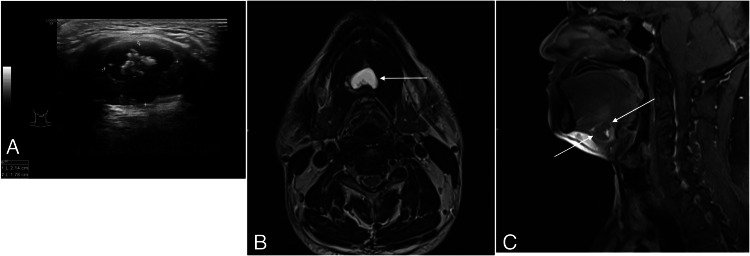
(A) US transverse image through the right mid thyrohyoid region shows a well-defined complex cystic lesion with a thick irregular wall and internal bright echogenic content which shows posterior shadowing. (B) Axial T2-weighted MRI of the neck shows a well-defined complex cystic lesion in the mid upper neck anterior to the thyrohyoid region with involvement of floor of mouth. (C) Sagittal T1 fat saturated post contrast (gadolinium) MRI of the neck shows a complex cystic lesion in the upper neck anterior to the thyrohyoid region with involvement of the floor of the mouth. It shows an enhancing thick irregular wall and a posteriorly situated enhancing nodule. US: Ultrasound, MRI: Magnetic Resonance Imaging
